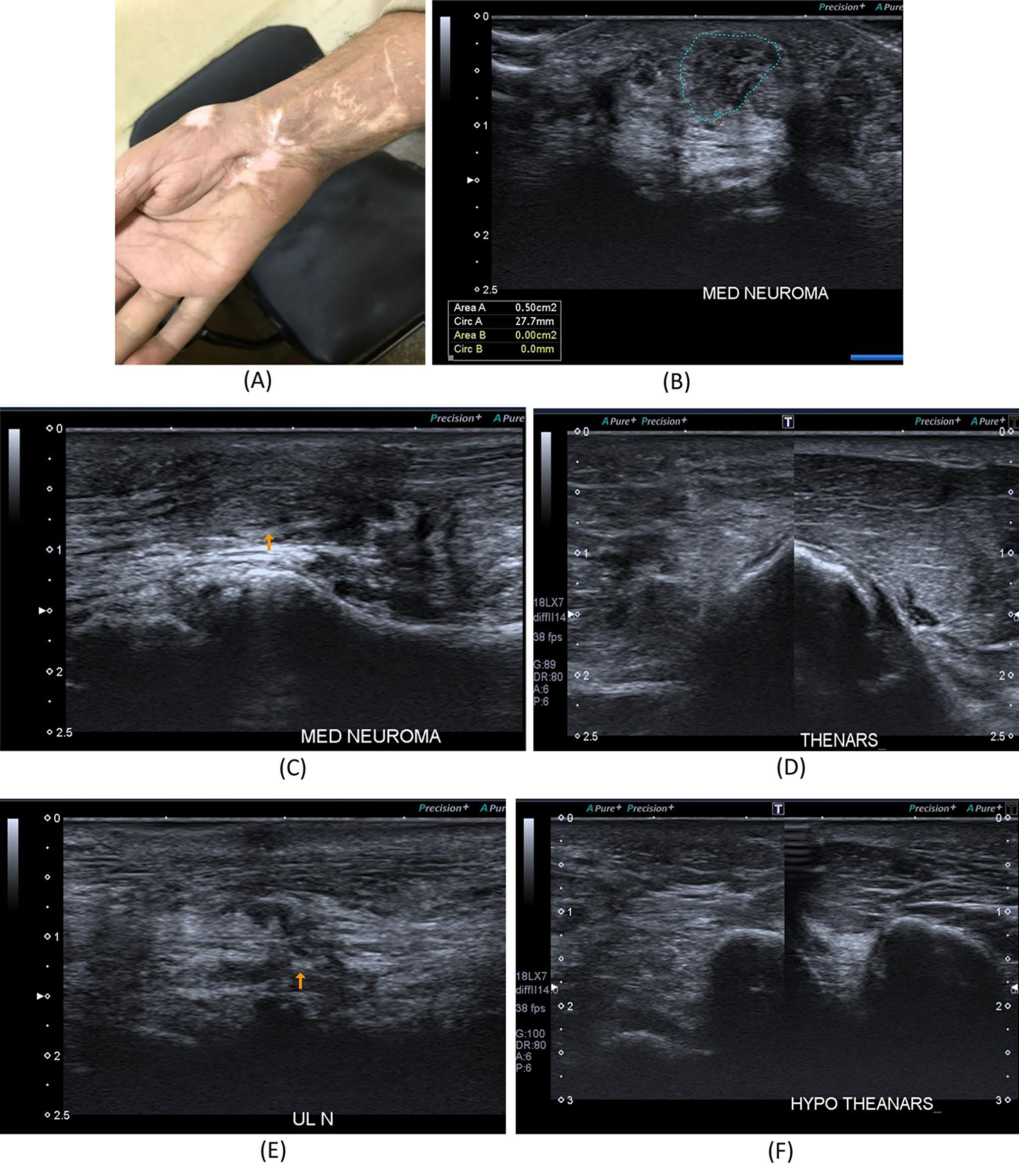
A 25-year- old male patient presenting with history of left wrist compression/degloving injury in 2020. He underwent tendon repair surgery. A Patient’s hand. B B-mode image, transverse axis, reveals thick hypoechoic swelling of the left median nerve with loss of fascicular architecture. C B-mode image, longitudinal axis, thickened, hypoechoic focal area with loss of fascicular architecture of the median nerve at the site of injury. D B-mode image of the thenar muscles, reveal loss of normal sonographic appearance of the left side muscles with increased echogenicity as compared to the right side. E B-mode image, longitudinal axis, shows discontinuous, disorganized left ulnar nerve at the distal forearm with related hypoechoic scar tissue formation. F B-mode image of the hypothenar muscles, reveal loss of normal sonographic appearance of the left side muscles with increased echogenicity as compared to the right side
